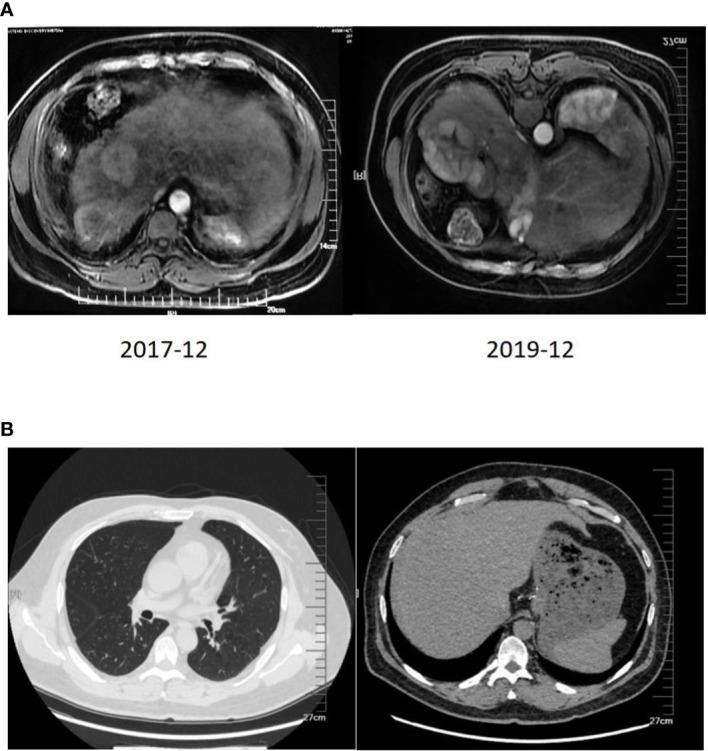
(A) Changes between the prior-treatment and post-treatment MRI pictures of the abdomen. (B) CT scan of the chest and abdomen one year after liver transplantation.
